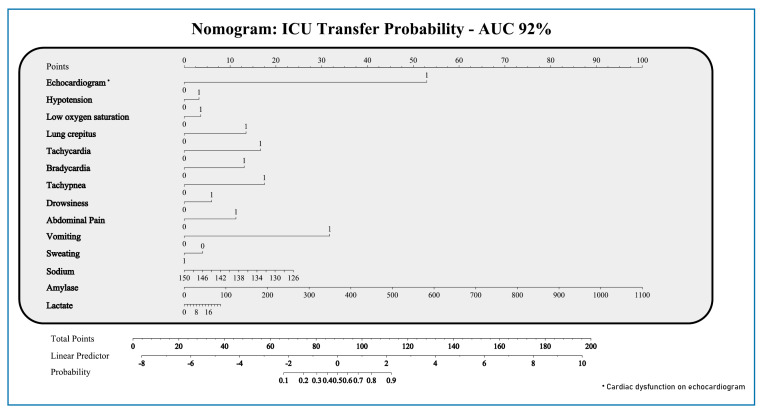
Preliminary ICU transfer prediction nomogram. *Cardiac dysfunction on echocardiography.
